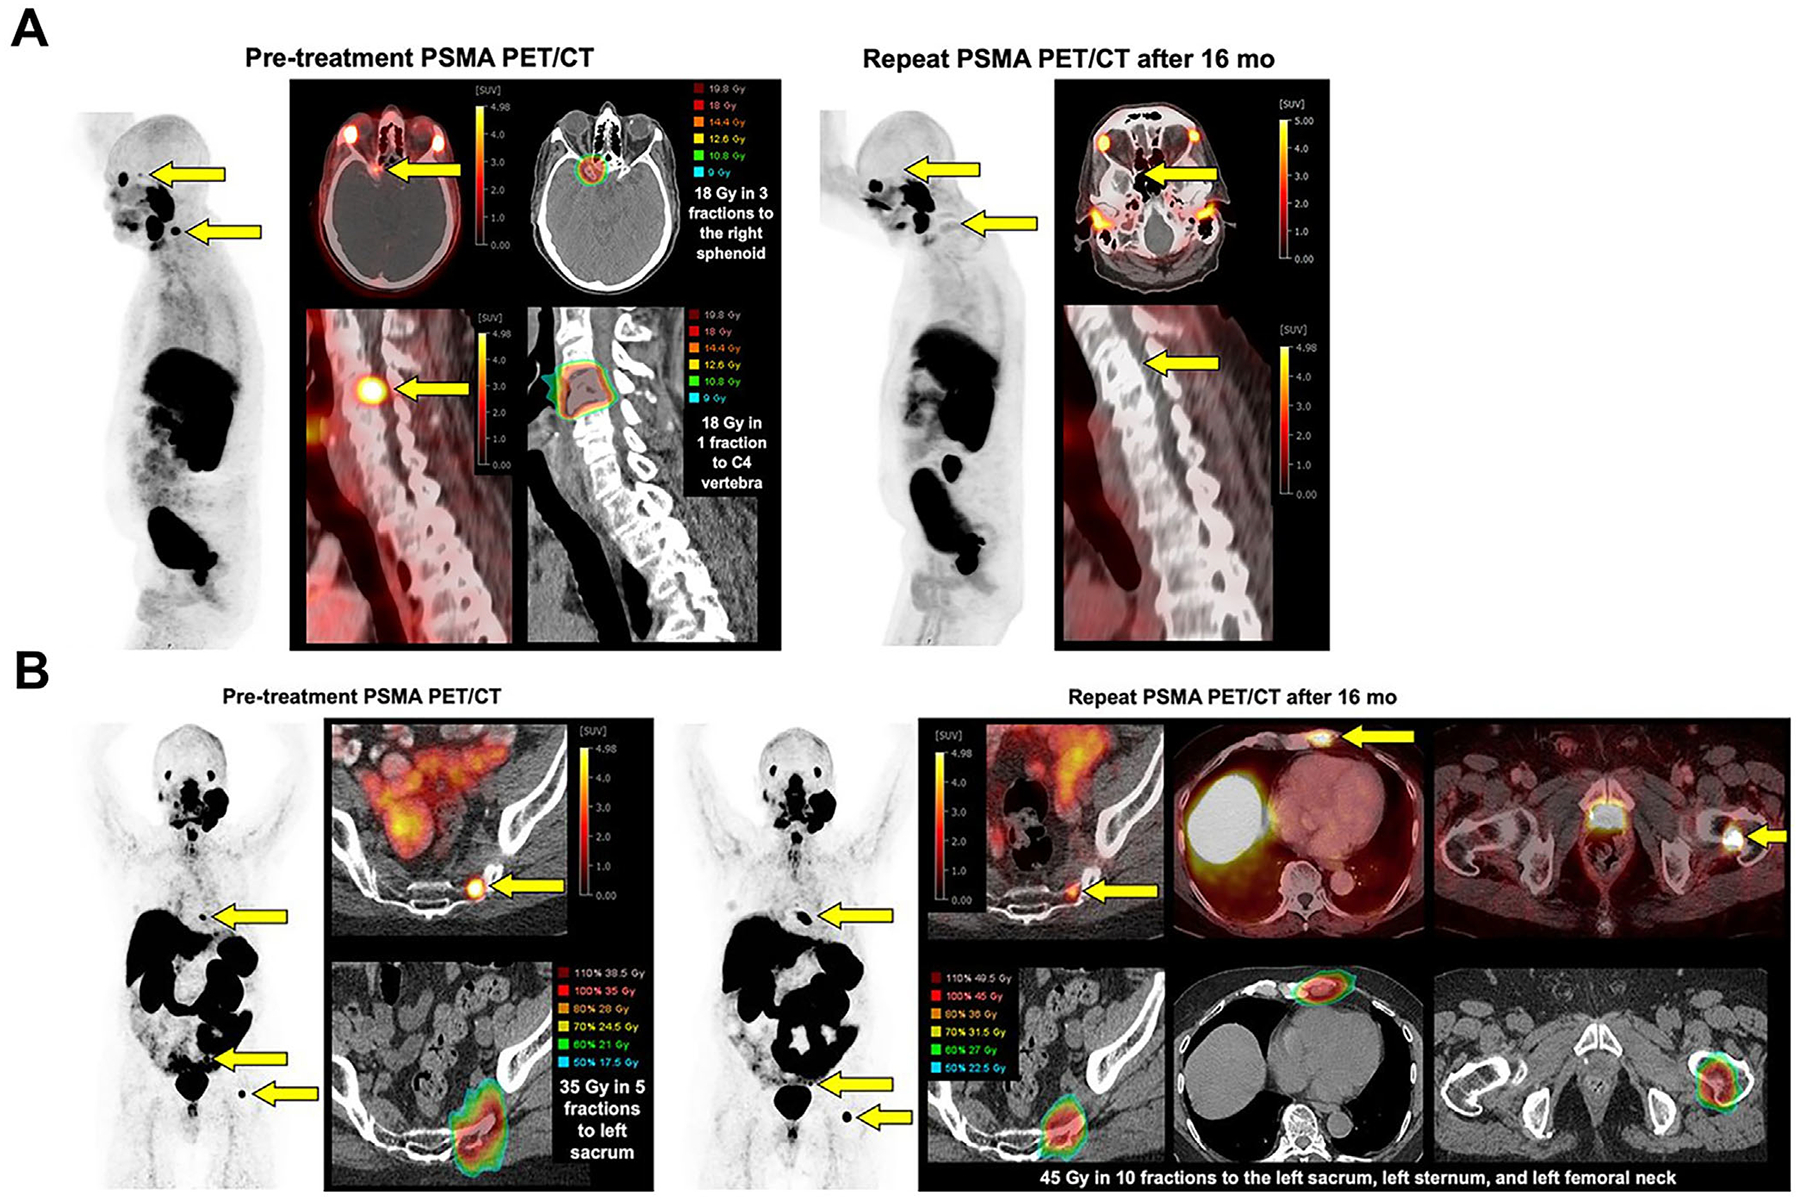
Sequential prostate-specific membrane antigen (PSMA) positron emission tomography (PET)/computed tomography (CT) imaging of metastatic prostate cancer before and after targeted radiotherapy. (A) New metastases in the C4 vertebra and right sphenoid bone (arrows) on baseline imaging, with follow-up showing resolution after metastasis-directed radiotherapy but subsequent multifocal progression. (B) New left sacral metastasis with stable lesions in the left femur and sternum (arrows). Follow-up imaging shows in-field failure in the sacrum and new progression in the left femur and sternum after initial radiotherapy. Reproduced from Ref. 92 with permission from the Society of Nuclear Medicine and Molecular Imaging, copyright 2024.
